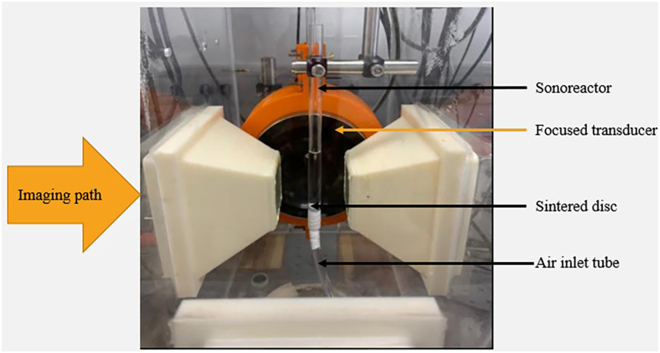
The ultrasound reactor for optimization experiments, showing the relative positioning of the transducer, sonoreactor, and imaging path within the sonoptic tank3.Connect the transducer to the waveform generator and power source, according to Figure 4.a.Connect the waveform generator via Channel 1 to a power amplifier.b.Link the outwards connection of the amplifier to the focused ultrasound transducer via an impedance matching network.c.Link the sync-out of the waveform generator to the delay generator.d.Attach both the high-speed camera and the passive cavitation detector (via an oscilloscope) to the delay generator across two trigger relays.
